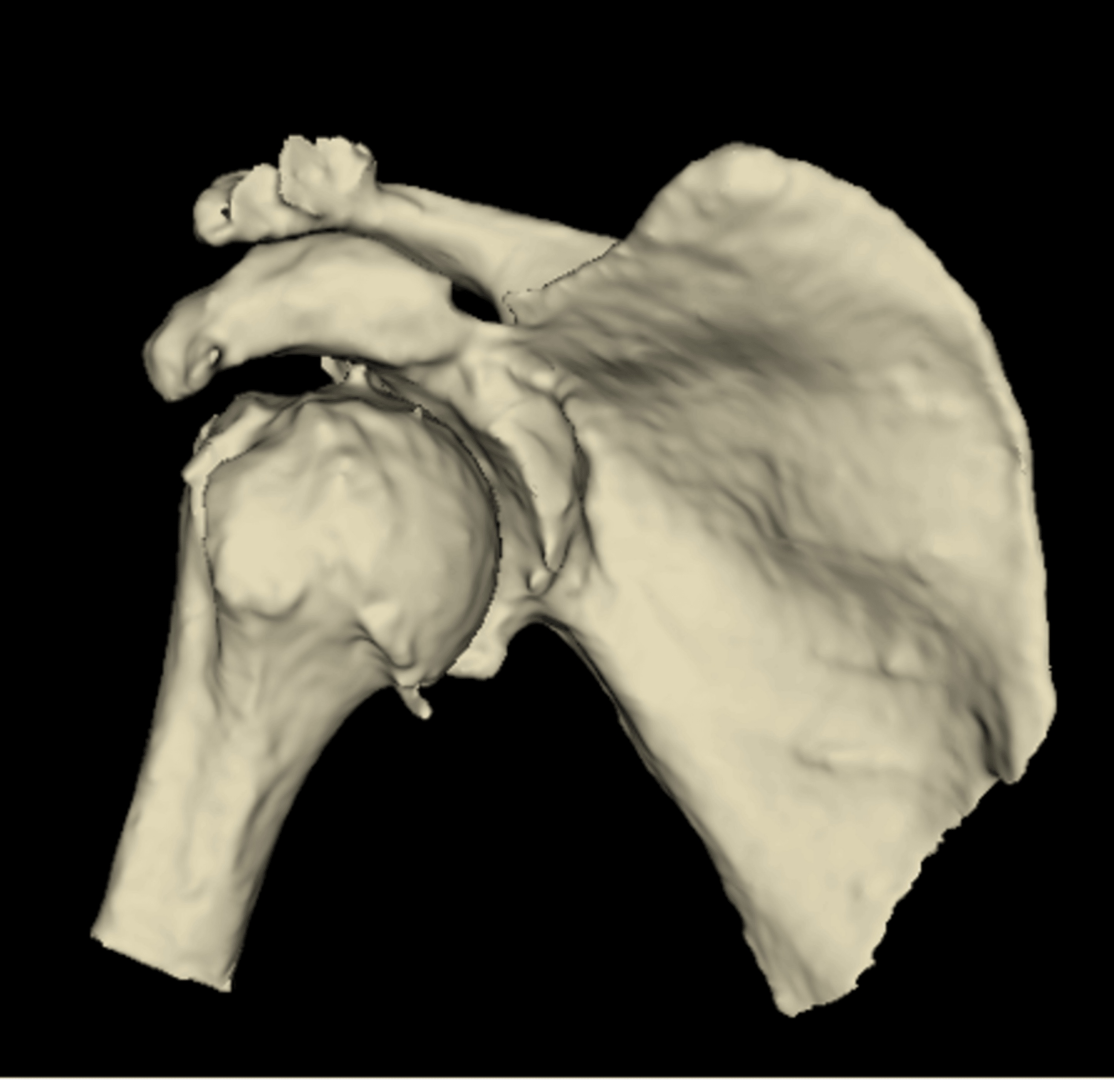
Pre-operative 3D CT coronal view of the glenohumeral joint demonstrating a Hill-Sachs defect.
